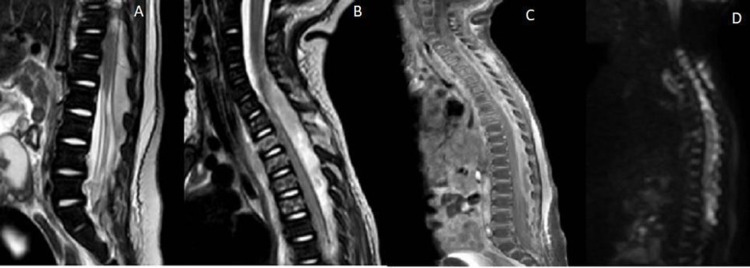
T2 Sagittal MRI of the thoracolumbar (A) and cervicothoracic (B) regions demonstrate posterior epidural collection causing significant cord compression. Post-contrast (C) and DWI (D) imaging confirm the diagnosis of spinal epidural abscess. MRI: magnetic resonance imaging; DWI: diffusion-weighted imaging
